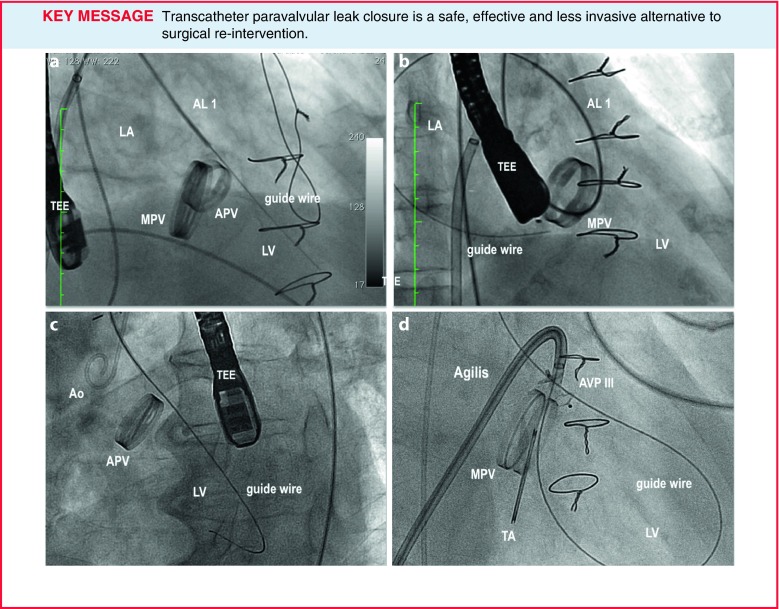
Technical approaches to TPVL closure. a Mitral PVL closure using an antegrade transseptal approach. b Mitral PVL closure using a retrograde approach. c Aortic PVL closure using a retrograde approach. d Mitral PVL closure using a deflectable sheath. *APV* aortic prosthetic valve, *MPV* mitral prosthetic valve, *Ao* Aorta, *AL* Amplatzer left, *LA* left atrium, *LV* left ventricle, *AVP* Amplatzer Vascular Plug, *TEE* transoesophageal echocardiography, *TA* tricuspid annuloplasty
